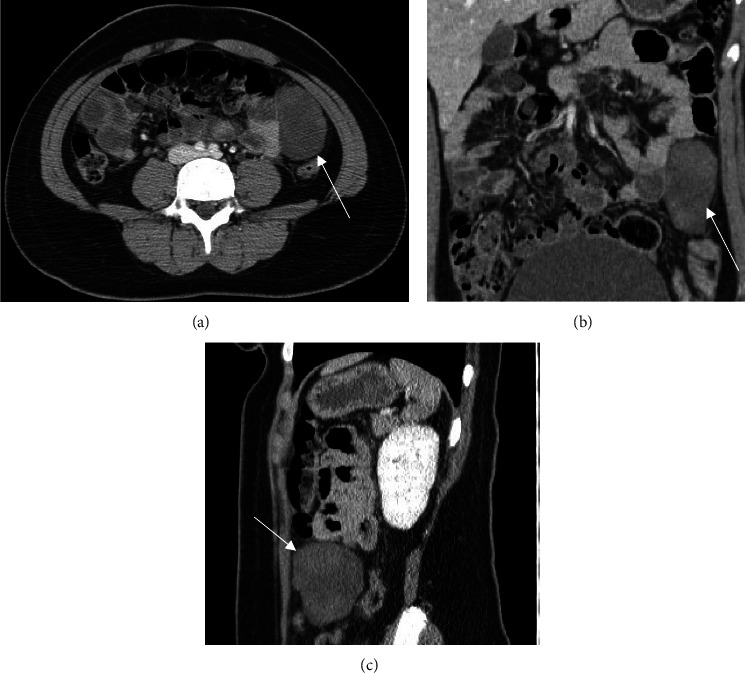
Axial (a), coronal (b), and sagittal (c) view of a contrast-enhanced CT of abdomen which showed a 9 × 5.5 × 3.9 cm smoothly marginated heterogenous hyperdense lesion in the left mid abdomen separate from the adjacent bowel loops (arrows).
